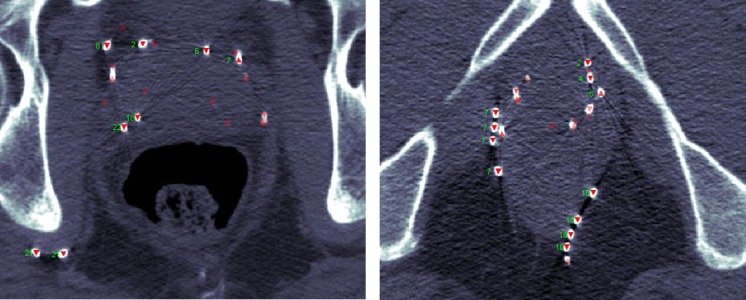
Day 30 axial computed tomographic images showing seed migration to unexpected locations
